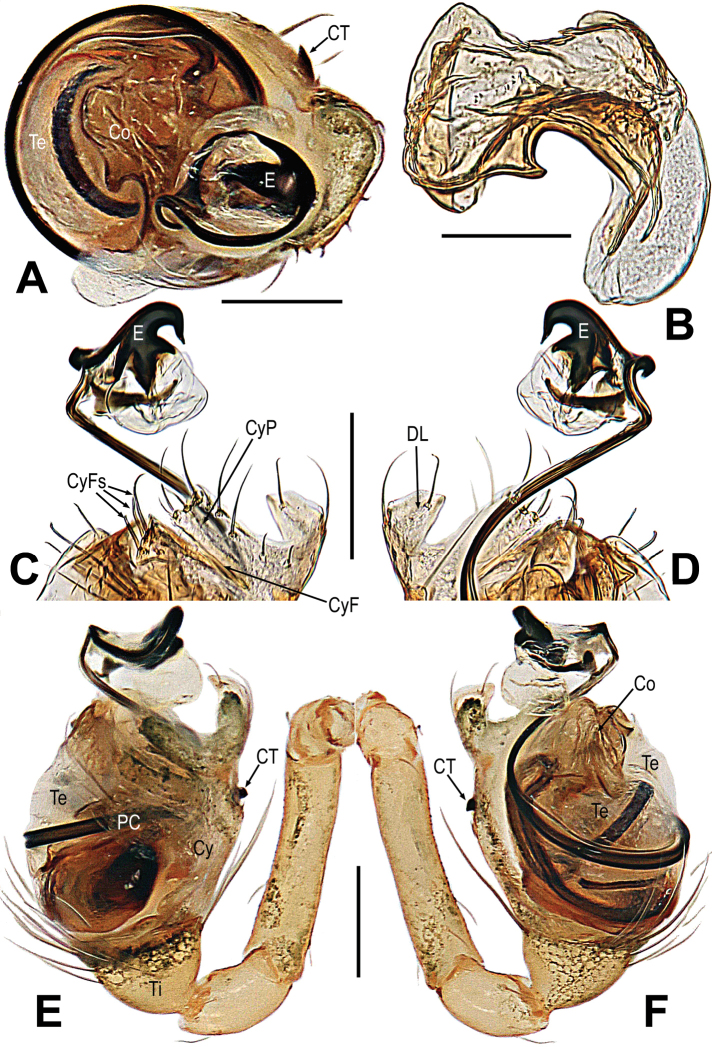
Microdiponeamenglunensis*A, E, F male palp B conductor C, D embolus and cymbial terminal A apical B dorsal C, E prolateral D, F retrolateral. Abbreviations: C = conductor; CT = cymbial tooth; Cy = cymbium; CyF = cymbial fold; CyFs = setae on cymbial fold; CyP = cymbial process; DL = distal lobe on cymbium; E = embolus; PC = paracymbium; SD = spermatic duct; Te = tegulum; Ti = palpal tibia. Scale bars: 0.10 mm (A, C–F); 0.05 mm (B).
